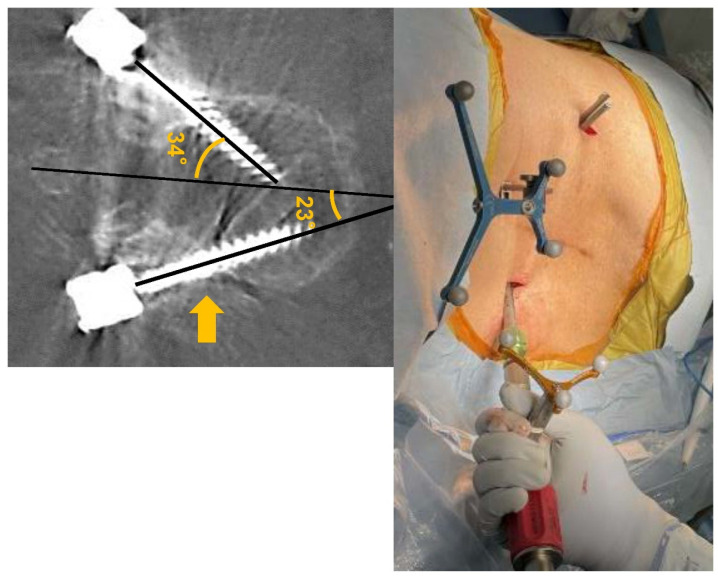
Axial CT and photographs of a representative case of the PPS downside placement in the lateral position; attention was needed to avoid a lateral breach. PPS, percutaneous pedicle screw. Yellow arrows indicate deviation outside of screw.
